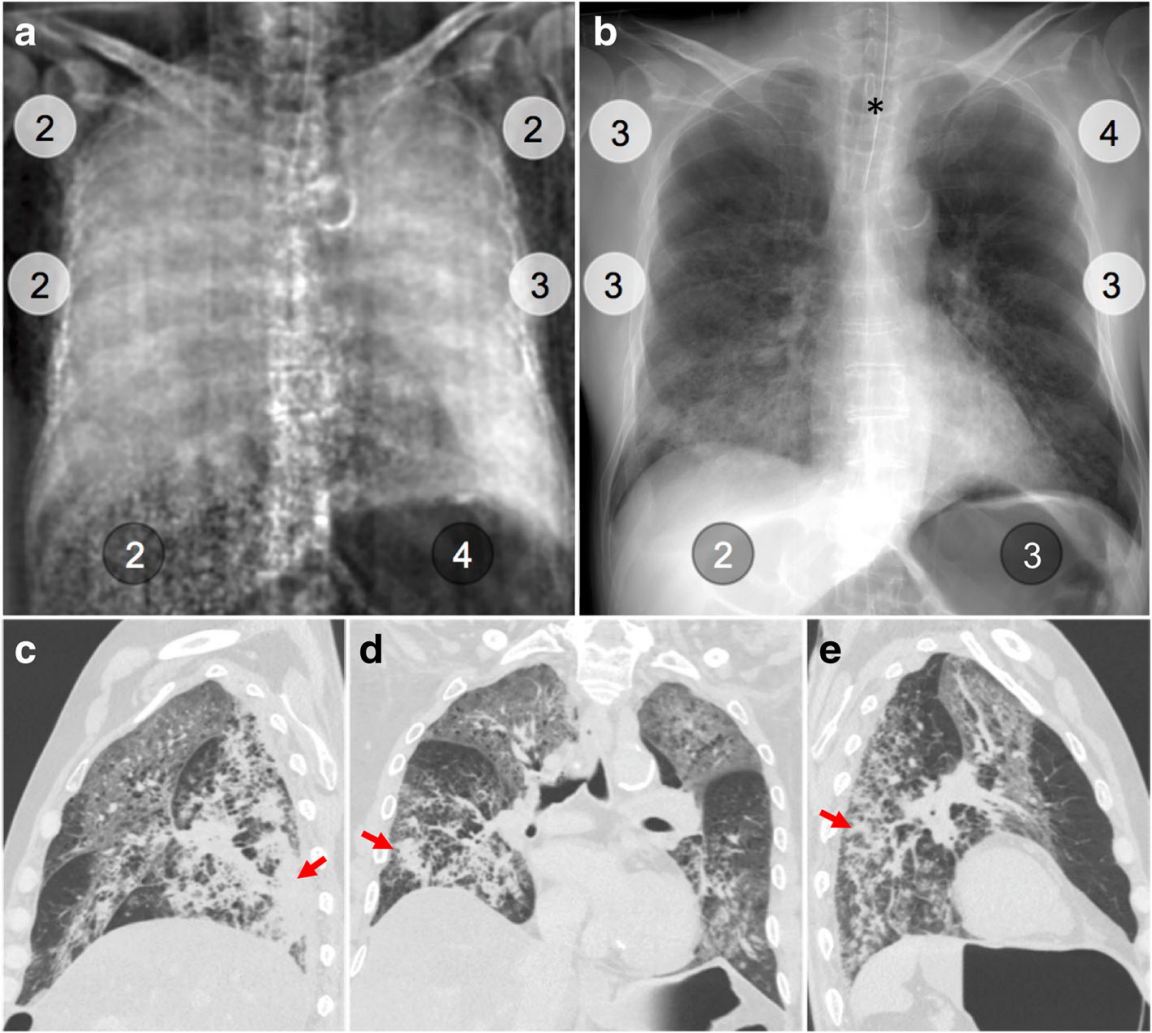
Dark-field chest radiograph, conventional x-ray, and CT of human body 6. Median visual grading (encircled numbers) of x-ray dark-field chest radiograph (a) by three independent readers shows markedly reduced dark-field signal in middle and lower zones of the right lung. Median visual grading of transmission in conventional x-ray (b) shows reduced transmission in the right lung upper zone as well as right and left lung middle zones and is lowest in right-lung lower zone. Sagittal (c, e) and coronal (d) CT images show interstitial and alveolar infiltrates in the right upper lobe, left upper lobe, and right middle lobe, as well as extensive pulmonary consolidation (red arrows) in the right lower lobe and, to a lesser extent, in left lower lobe. Asterisk marks the location of the endotracheal tube
